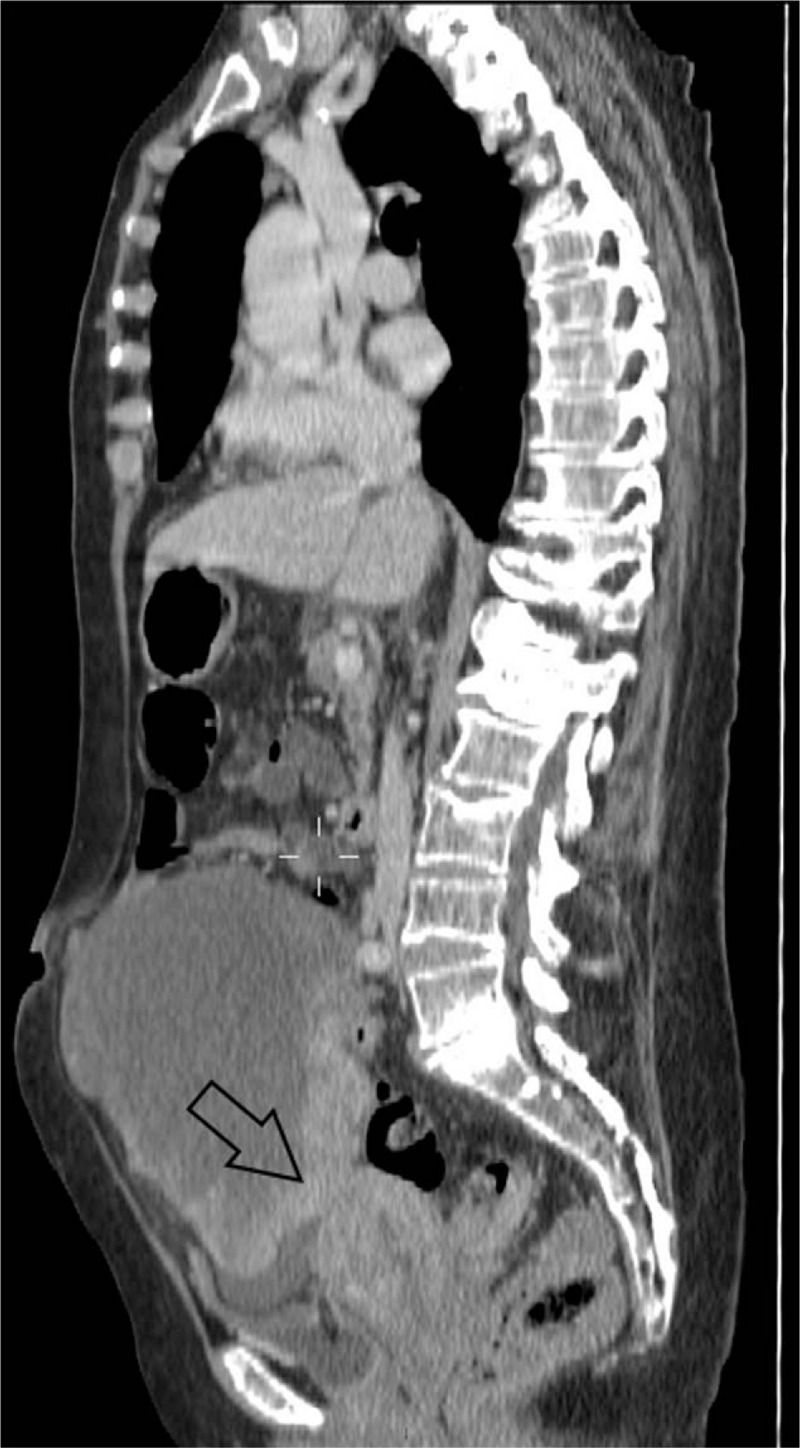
Computed tomography (CT) scan. Loss of the fatty plane between the mass and the uterus (black arrow).
